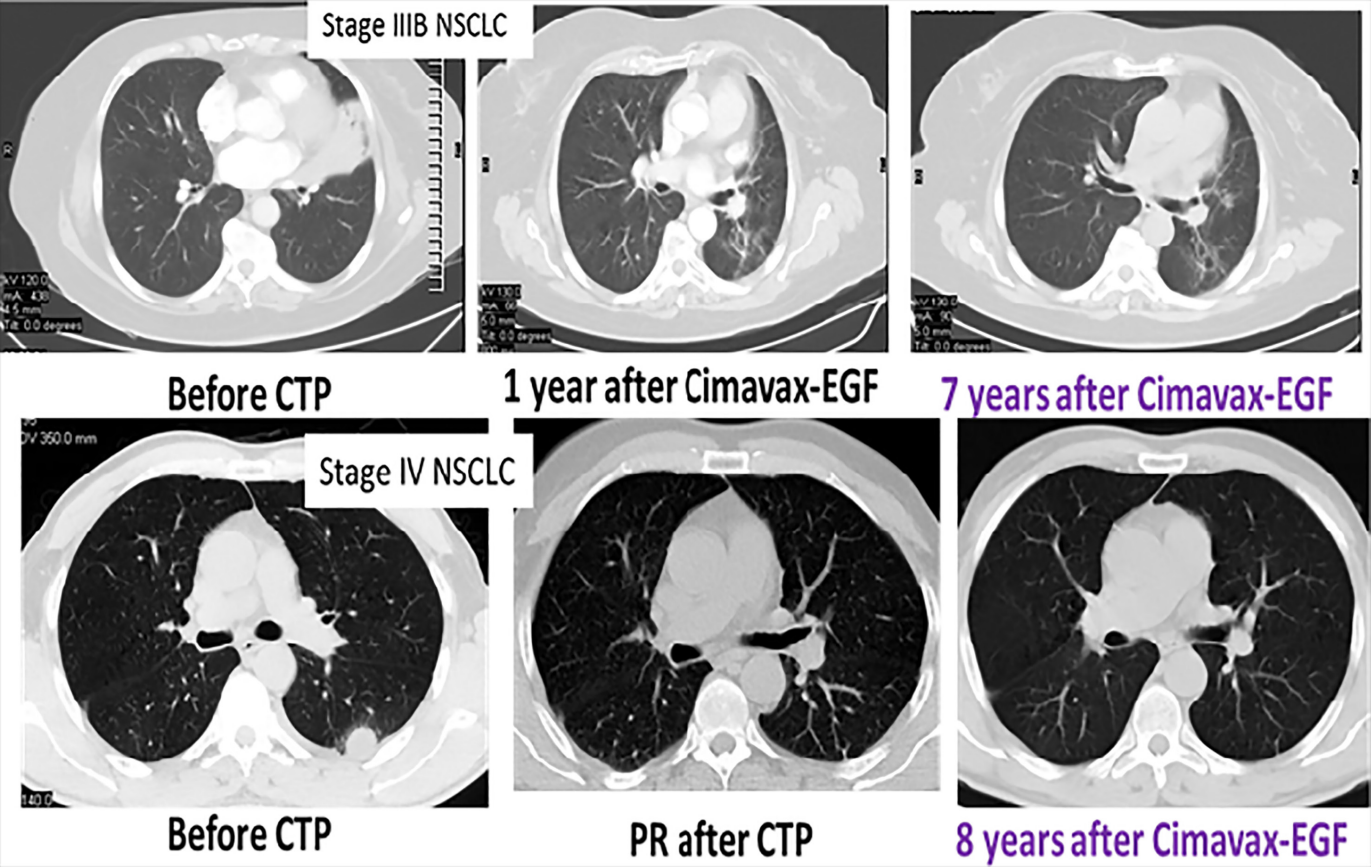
Long-lasting disease control after CIMAvax-EGF. CT scan series of two representative patients.
